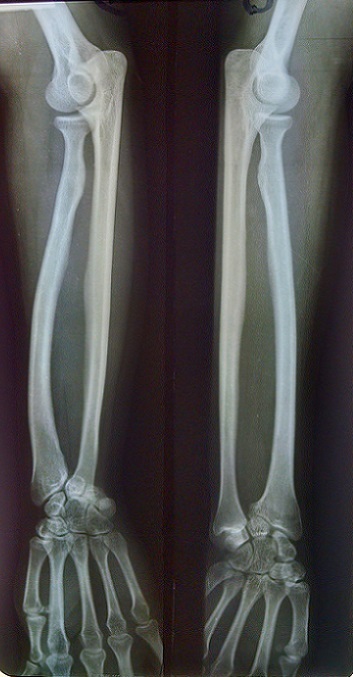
Radiographie comparative de l'avant-bras vue de face d'une déformation de Madelung
